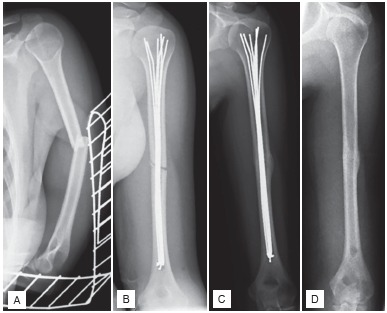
(A) Anteroposterior radiograph of a 31 year old female with an AO type 12-A3 mid-diaphyseal fracture of the humerus; (B) Postoperative radiograph showing complete filling of the medullary cavity with the elastic nails; (C) Follow-up at 10 months after surgery shows bony union; (D) Follow-up at one year after implant removal.
